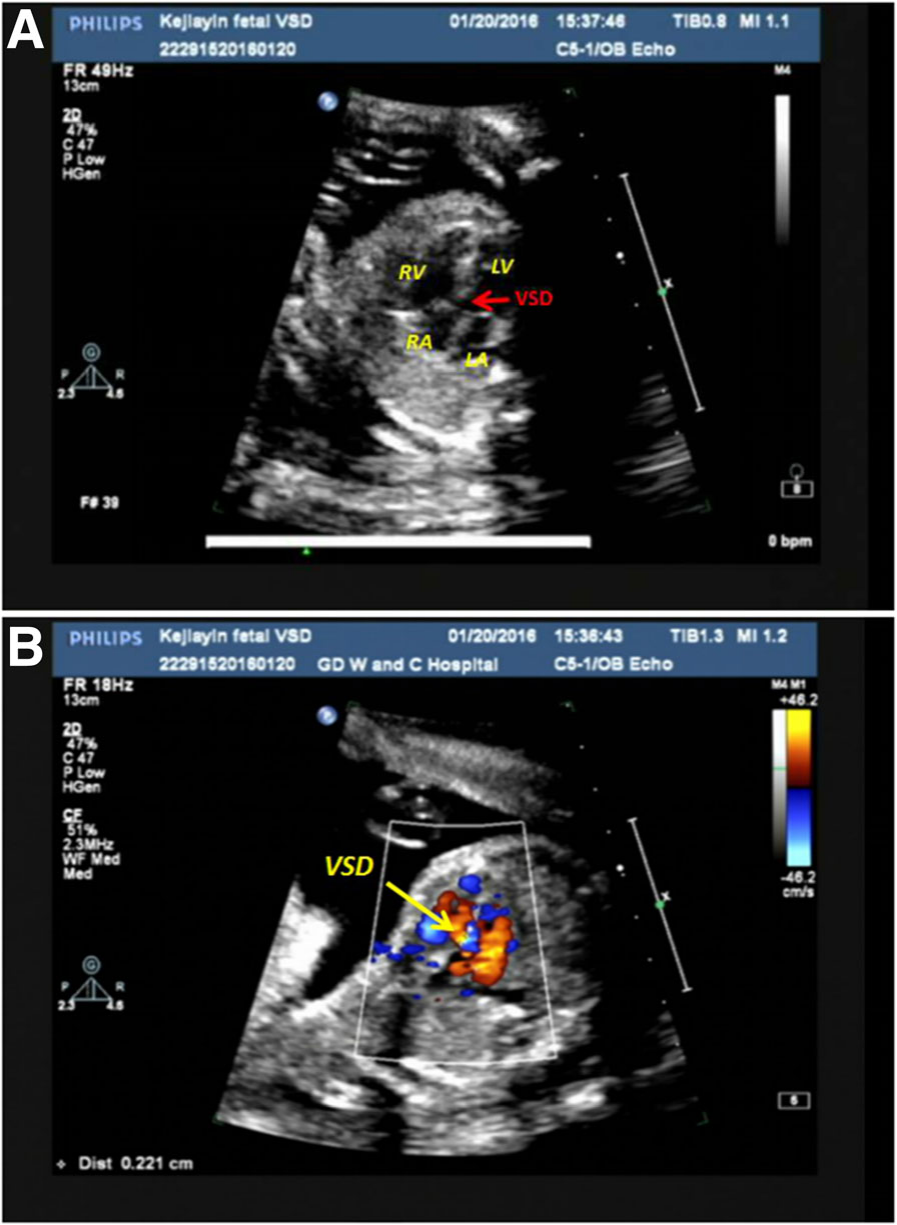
Ultrasound examination images. a Ultrasound examination result at 30wk. A ventricular septal defect for 2.2 mm was shown as the arrow in the image. Abbreviations: LA, left atrium; LV, left ventricle; RA, right atrium; RV, right ventricle; VSD, ventricular septal defect. b Ultrasound examination result at 33wk. A ventricular septal defect (VSD) for 3.0 mm was shown as the arrow in the image
